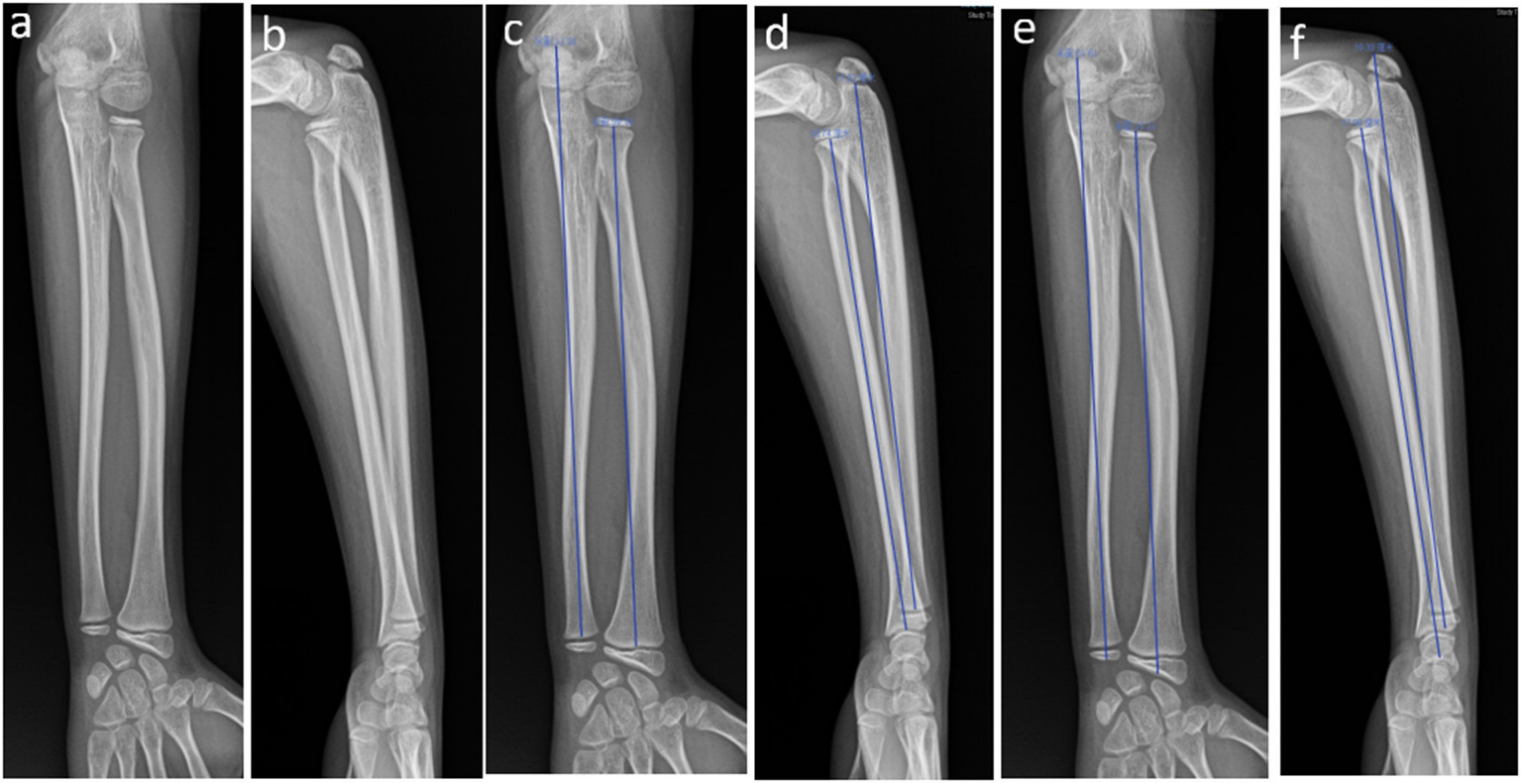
Anterior–posterior (AP) and lateral (LAT) radiographs of the left radius and ulna in a 10-year-old girl. (a) AP of the left forearm; (b) LAT of the left forearm; (c) AP showing measurement of the SLR and SLU; (d) LAT showing measurement of the SLR and SLU; (e) AP showing measurement of the TLR and TLU; (f) LAT showing measurement of the TLR and TLU.
